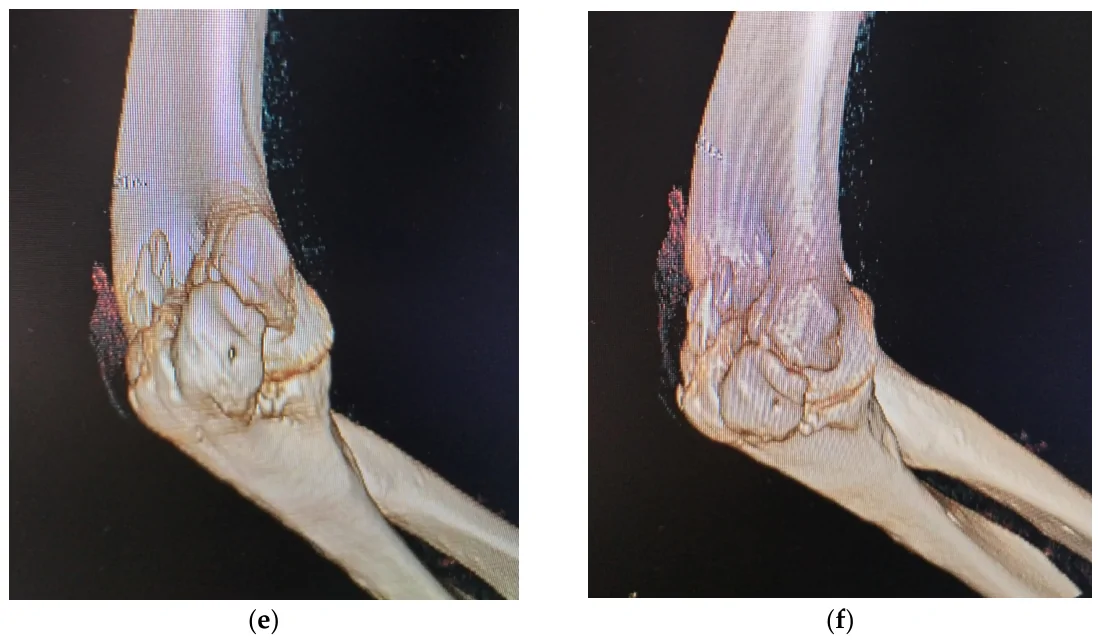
X-ray (a) fracture of olecranon, (b) osteosynthesis with a plate and screws, (c,d) secondary periarticular ossification, (e,f) CT findings.
